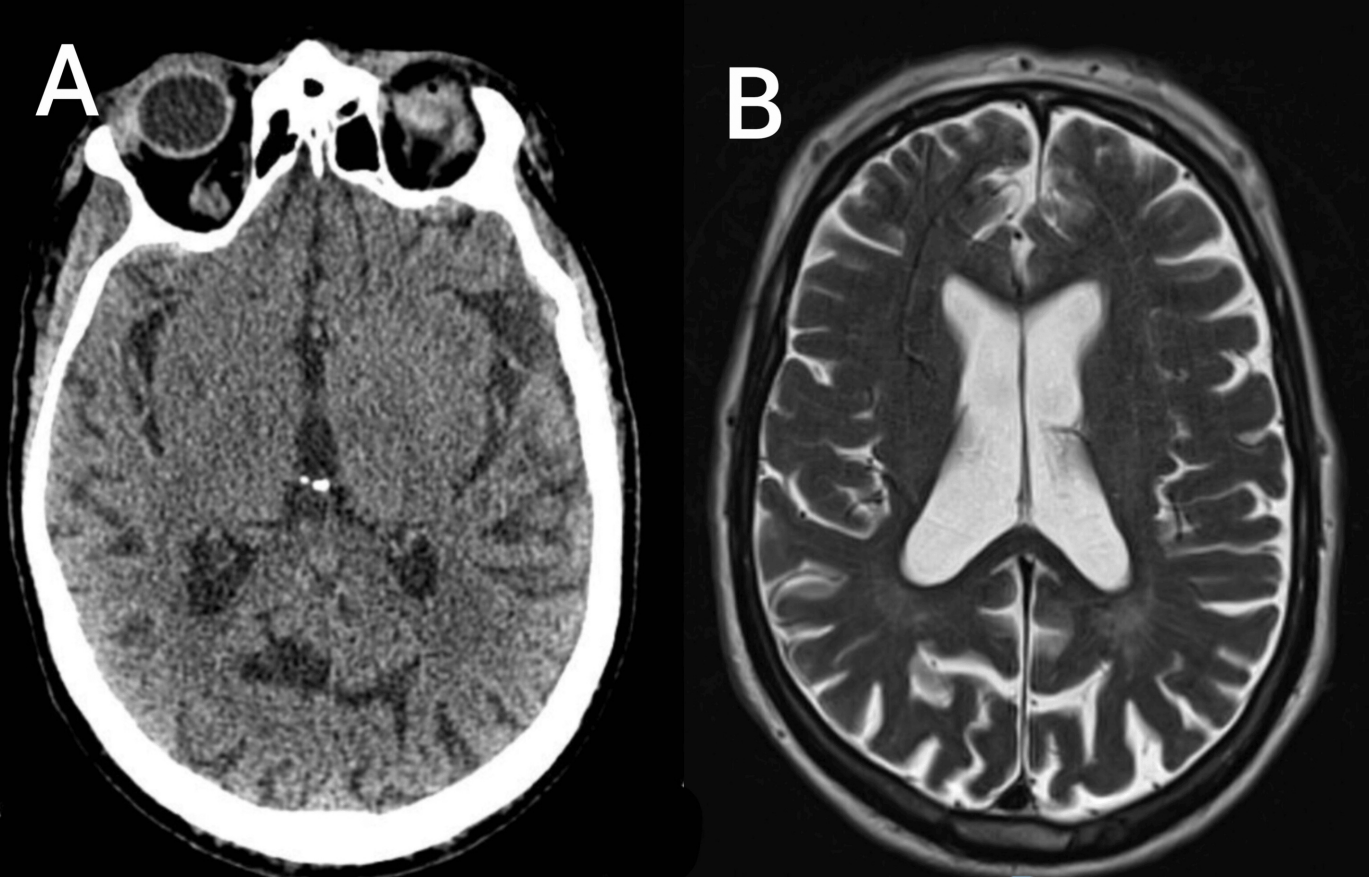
CT and MRI brain of the patient showing no significant abnormality. (A) A section of CT brain of the patient; (B) A section of MRI brain of the patient.
